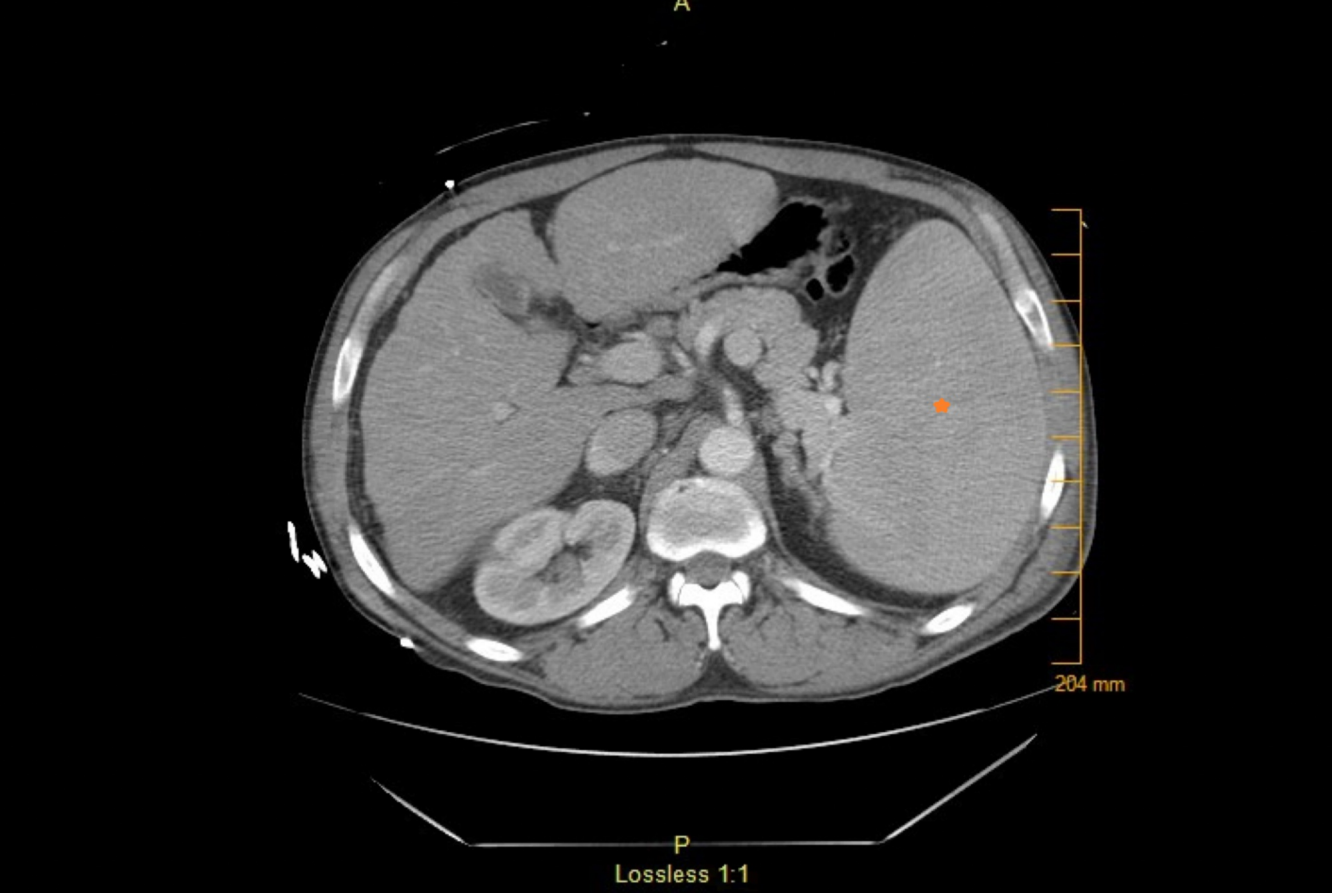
CT thorax with contrast capturing part of the abdominal cavity showing splenomegaly marked with the orange star. CT: computed tomography
